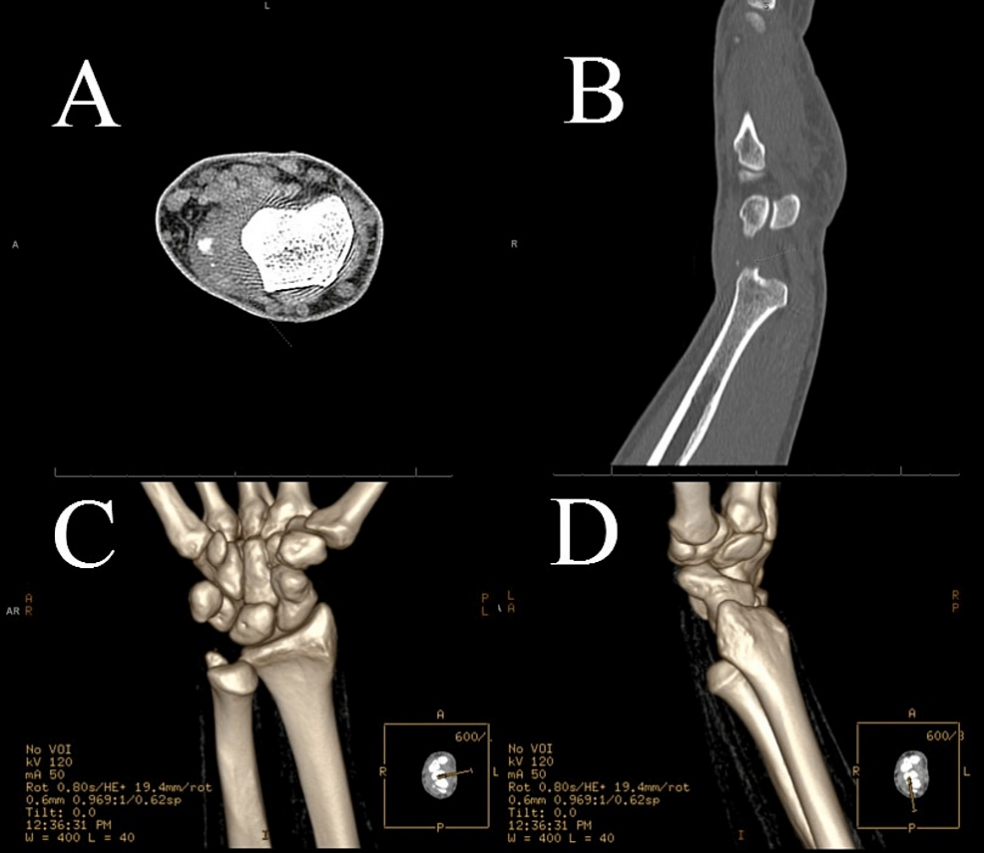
Musculoskeletal CT axial, sagittal, and three-dimensional images of the right hand and wrist. (A and B) Axial and sagittal views via musculoskeletal CT of the right hand and wrist. (C and D) Three-dimensional images via musculoskeletal CT of the right hand and wrist. These images were initially interpreted as “a small calcific density along the volar aspect of the ulna styloid which may represent avulsion fracture fragment.” The addendum later notes a “volar dislocation of the ulna with punctate avulsion fracture fragment along the dorsal aspect at the level of the ulnar styloid.” CT: computed tomography.
